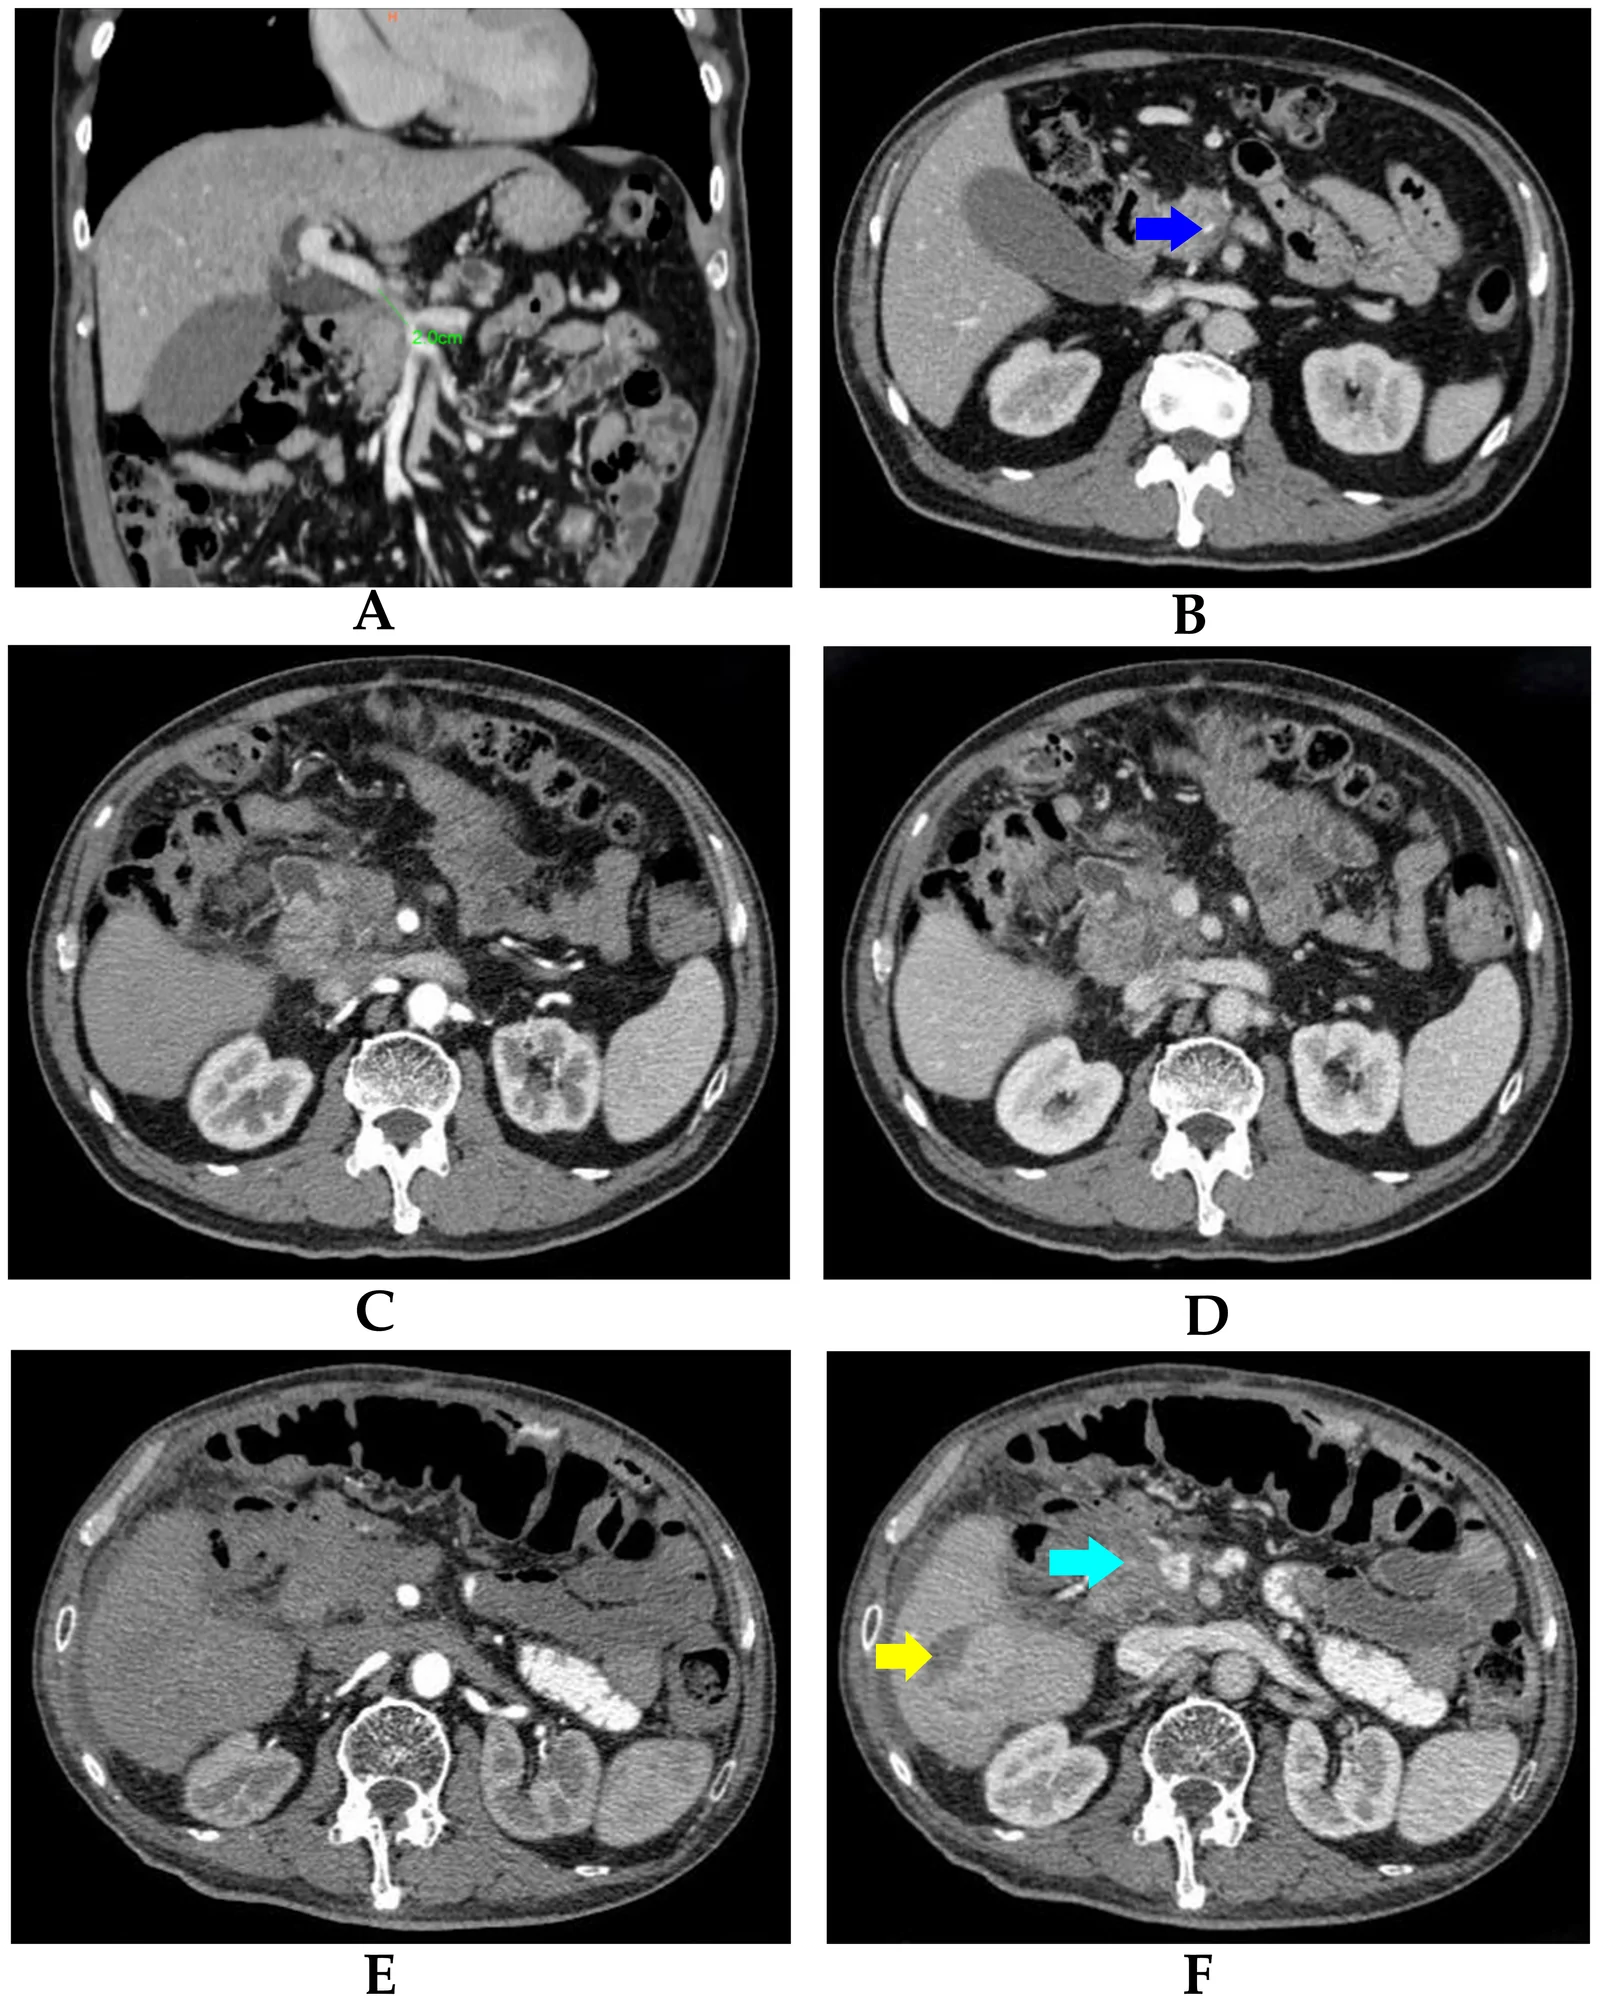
Longitudinal contrast-enhanced CT findings across the treatment course. (A, B) Contrast-enhanced CT images obtained before the initial IRE procedure in January 2021. (A) Coronal image showing approximately 2.0 cm of craniocaudal venous involvement at the superior mesenteric vein–portal vein (SMV–PV) confluence. (B) Axial image showing tumor involvement and marked narrowing of the SMV–PV confluence (blue arrow). (C, D) Arterial- and portal venous-phase contrast-enhanced CT images obtained during follow-up in August 2023, showing that the local pancreatic lesion remained relatively stable. (E, F) Arterial- and portal venous-phase contrast-enhanced CT images obtained in January 2026 after repeat pancreatic IRE and RFA of the hepatic metastasis. In (F), the cyan arrow indicates the pancreatic IRE-treated area, and the yellow arrow indicates the hepatic RFA-treated area.
